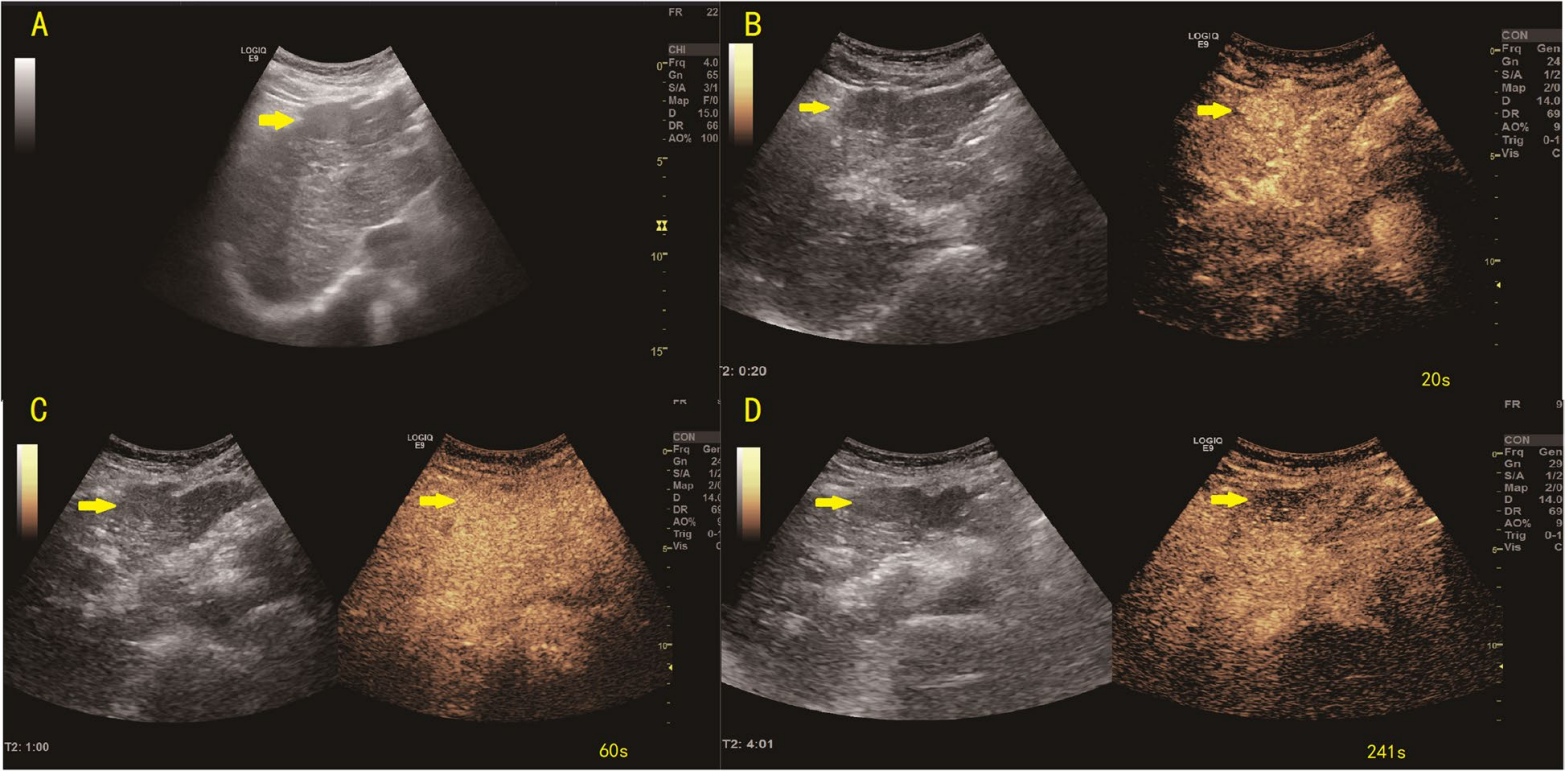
Typical HCC CEUS imaging. The mass is estimated to measure approximately 2.8 × 2.2 cm. A hypoechoic mass located in the left lobe (A). On CEUS, the mass displayed hyperenhancement after contrast agent injection (B). Contrast agent washout was observed as a isoenhancement at the lesion during the portal venous phase (60 s) (C) and hypoenhancing during the late phase (4 min 01 s) (D). The ‘Arterial phase hyperenhancement with whole showing marked washout in late in onset (60 s)’ lesion was classified as LR-5 based on the CEUS LI-RADS guidelines. CEUS = contrast-enhanced ultrasound; HCC = hepatocellular carcinoma; LI-RADS = Liver Imaging Reporting and Data System; LR = LI-RADS category
